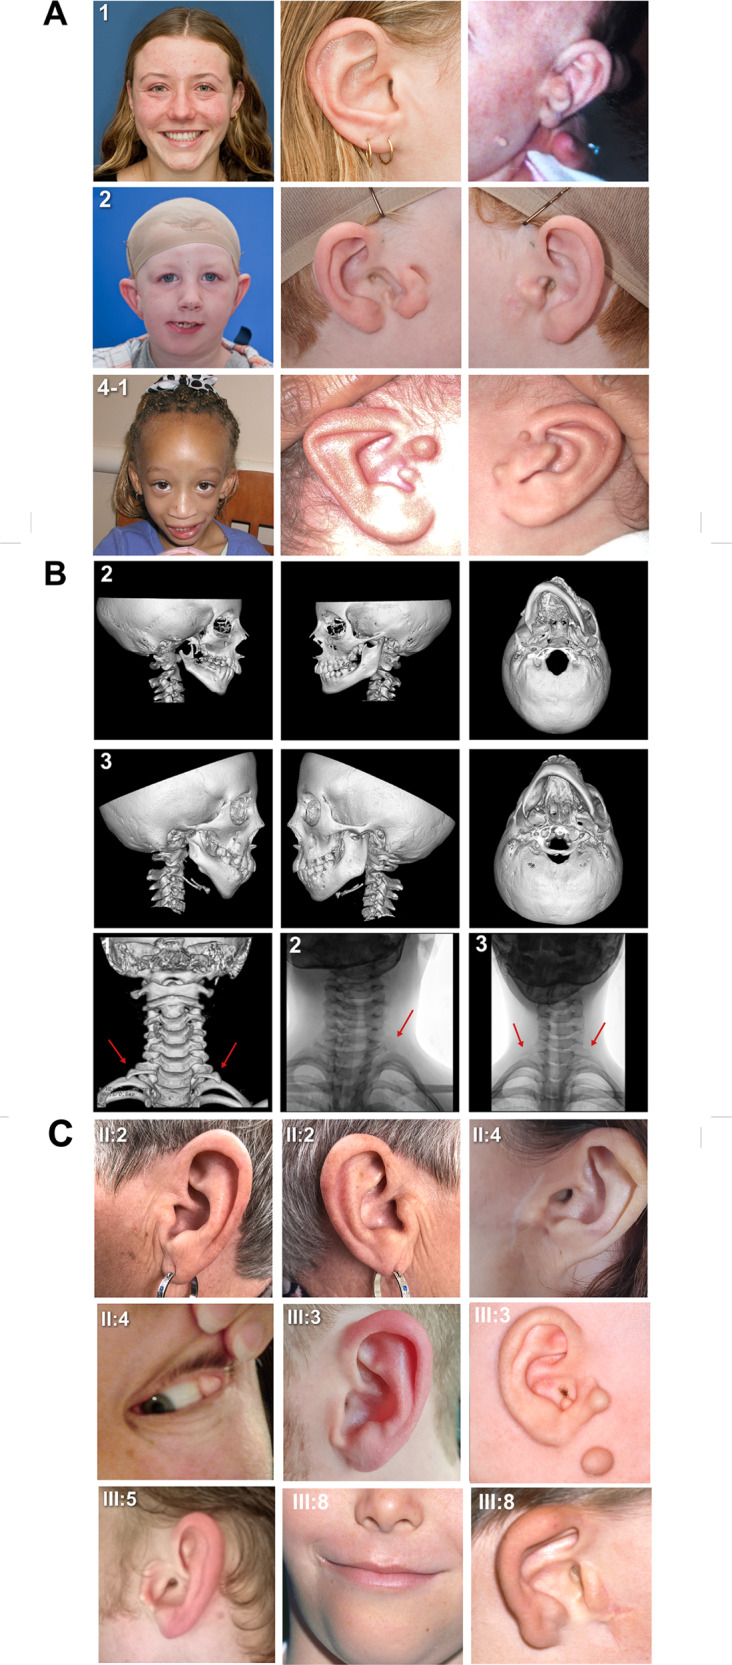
Clinical Images of Individuals with *SF3B2* haploinsufficiency. A. Photographs of three individuals demonstrating the shared phenotype. Proband 1 (c.2329dupG) demonstrates mandibular hypoplasia on the left, scar from preauricular skin tag excision on the right, and tragal duplication and facial skin tag on the left side prior to removal. Proband 2 (c.1780-2 A > -) demonstrates maxillary and mandibular hypoplasia on the right side in addition to bilateral tragal abnormalities. Proband 4-1 (c. 1608dupG) presented with maxillary and mandibular hypoplasia and tragal abnormality on the left side, and bilateral preauricular tags. B. Three-dimensional (3D) computed tomography (CT) reconstructions of the craniofacial skeleton of probands 2 and 3. Frontal, lateral, and submental views demonstrate maxillary and mandibular hypoplasia with resulting facial asymmetry. The third row demonstrates, from left to right, 3D CT scans of the cervical spine of Proband 1 and X-rays of Proband 2 and 3. Arrows in each figure indicate the cervical ribs. C. Photographs of family members with a shared *SF3B2* startloss variant (p.M1?) demonstrating variable external ear phenotypes. Images of II:2 demonstrate a right ear markedly smaller than the left. Images of II:4 demonstrate a tragal abnormality and scar from prior preauricular skin tag removal, in addition to an epibulbar dermoid. III:3 presented with bilateral tragal abnormalities and tags (right side ear photo from childhood). Images of III:5 show an underdeveloped tragus. III:8 had tragal abnormality, scar from tag excision, right lateral oral cleft, and chin deviation due to mandibular hypoplasia.
